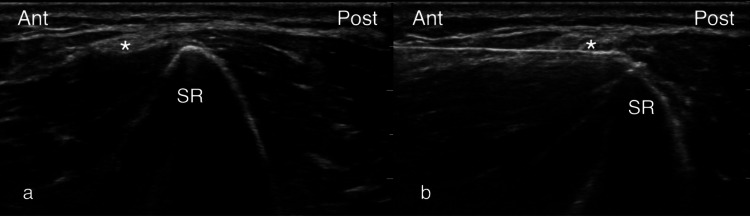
The placement of RF probe. (A) Sonographic transverse axis view at the level proximal to the lateral epicondyle, at which the contour of the humerus assumes a peak appearance at the SR. The PCNF-BrEpi (asterisk, *) is seen as a small caliber with several fascicles, located near the peak of the SR. (B) The RF probe is guided in-plane, in an anterior-to-posterior direction, to contact the undersurface of the PCNF-BrEpi. Ant: anterior; Post: posterior; RF: radiofrequency; SR: supracondylar ridge; PCNF-BrEpi: epicondylar branch of the posterior cutaneous nerve of the forearm
